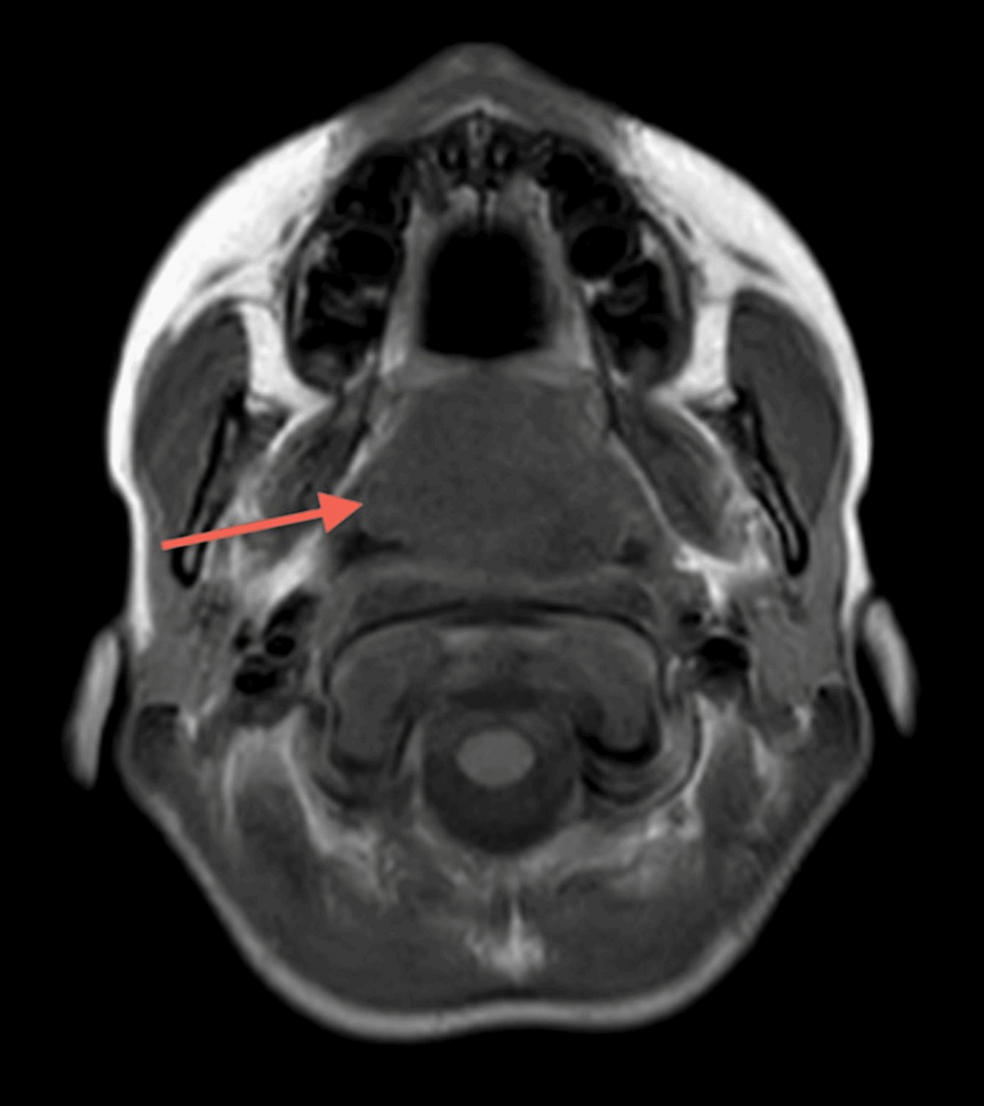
MRI Neck (T1) The large nasopharyngeal well-circumscribed cyst measures 47 x 28 x 35 mm. The sheer volume of this Thornwaldt cyst is remodeling the undersurface of the sphenoid sinus. The cyst is anteriorly eroding the posterior edge of the hard palate and midline vomer segment of the nasal septum, the uvula is displaced significantly into the oral cavity. Parapharyngeal recesses are preserved. No intracranial extension.
